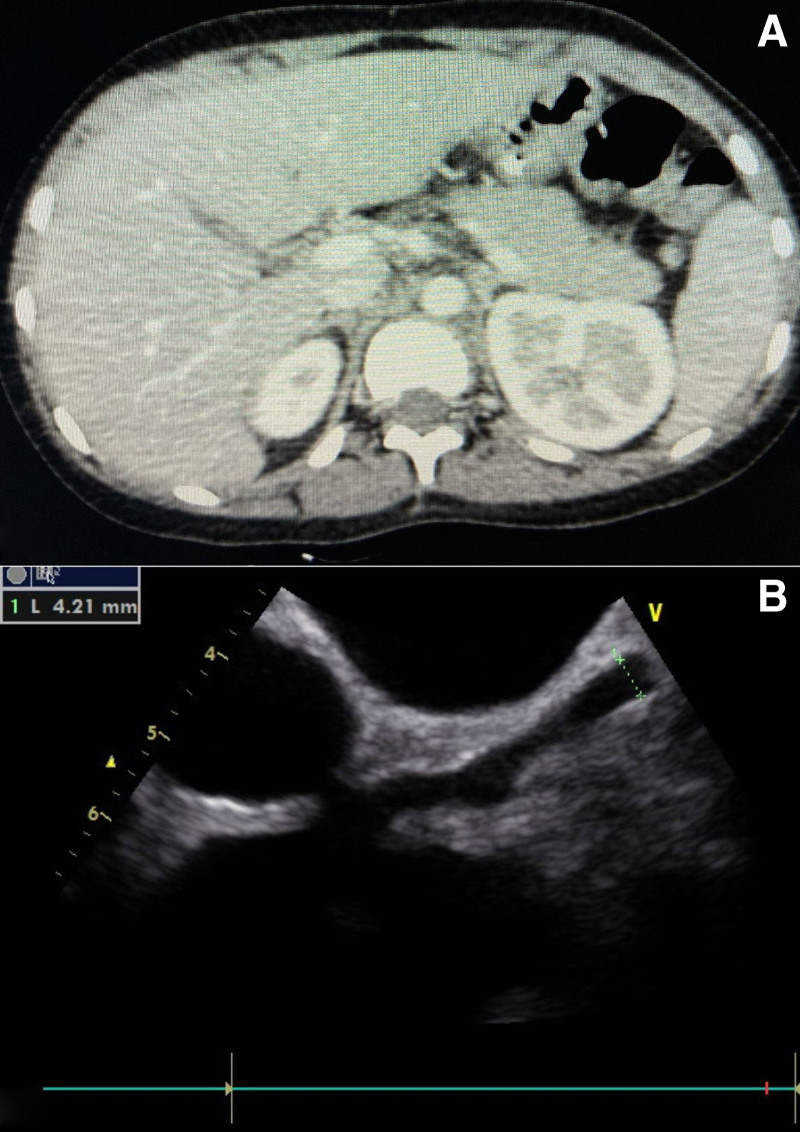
A) CT scan showing bulky pancreas, marked fat stranding around head, body and tail with peripancreatic collection. B) Echocardiography showing dilated LAD and lack of tapering in LAD. LAD = left anterior descending artery.
